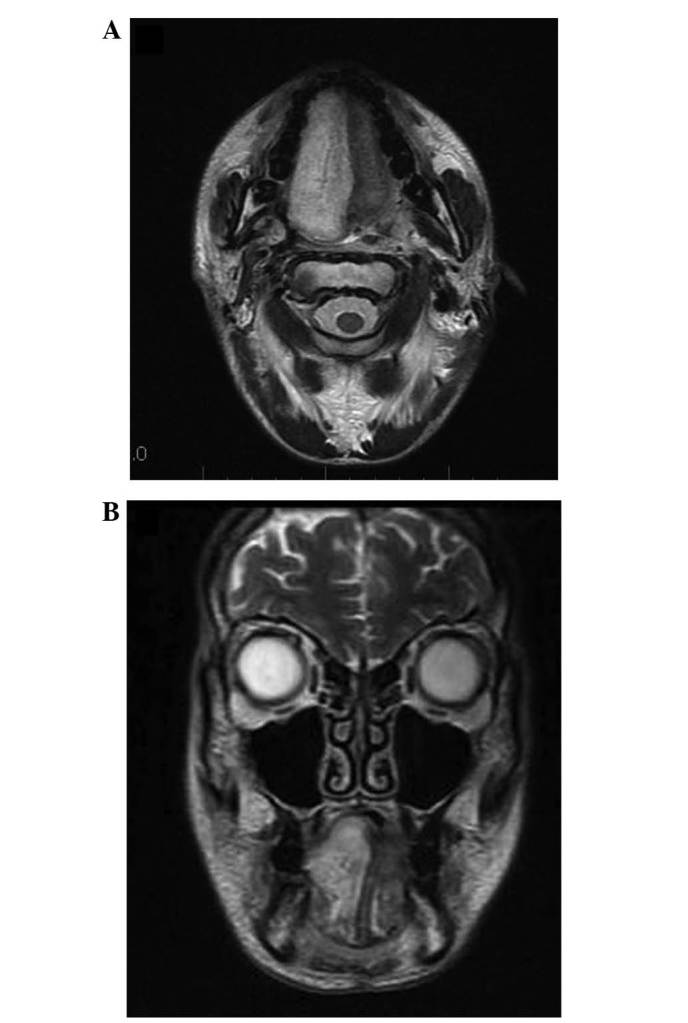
(A) MRI T2 axial scan revealed a suspected tumor recurrence on the right side of tongue. (B) MRI coronal scan of the tumor of the right side of the tongue. MRI, magnetic resonance imaging.
